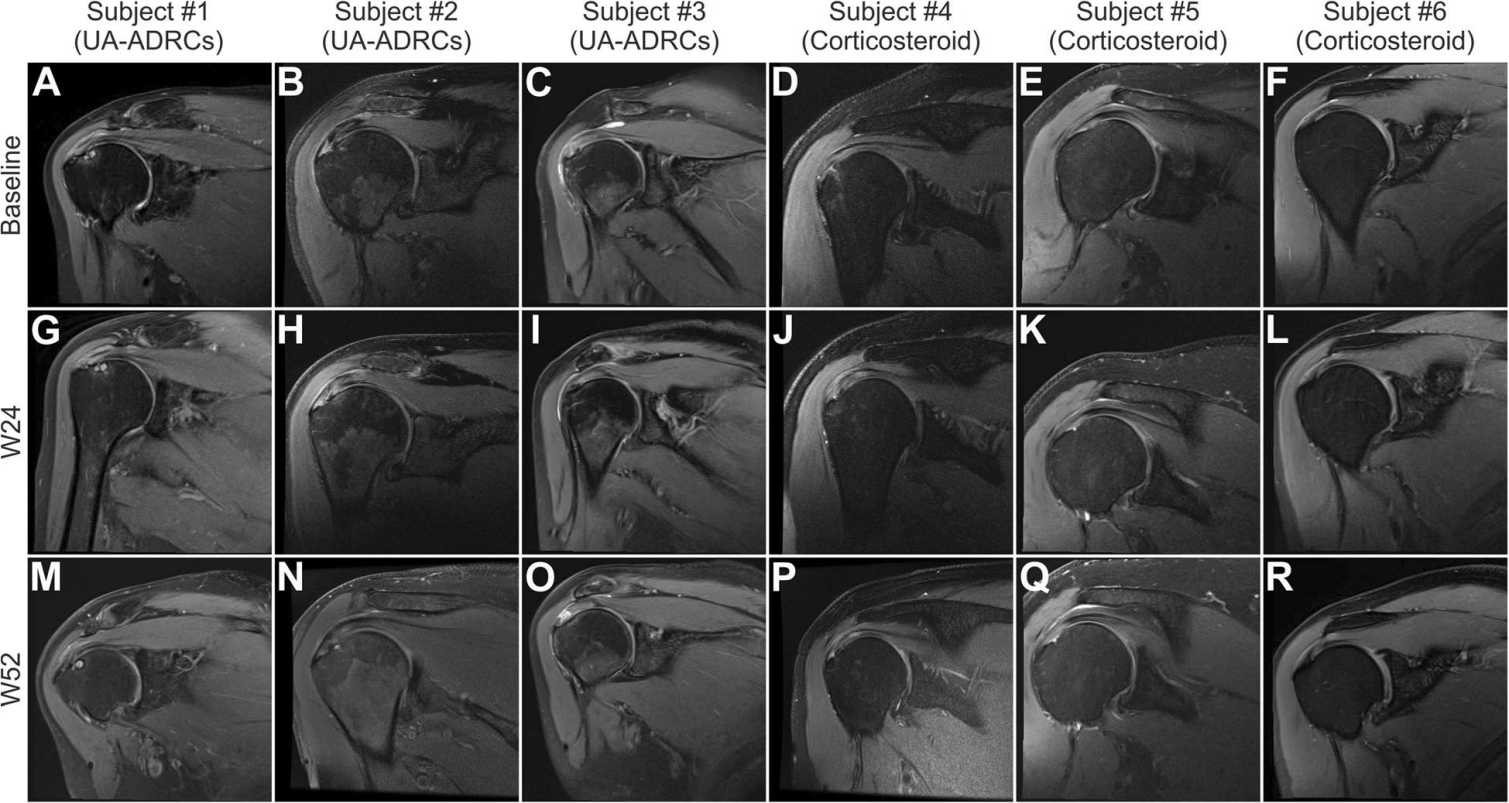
Coronal MRI scans (T2, proton density-weighted, fat-saturated (PD FS)) of the index shoulder of select subjects treated with respectively UA-ADRCs (a–c, g–i, m–o) or corticosteroid (d–f, j–l, p–r) in this pilot study. Repetition time (TR) was 2375 ms in n, 2950 ms in d, 3317 in j, 3500 in a, c, e, f, i, k, m, o, q, r, 3516 in p, 3660 in l, 3820 in g, and 3917 in b, h. Echo time (TE) was 34 ms in a, c, e, f, g, i, k–m, o, q, r, 37 in n, 45 in b, h, j, 46 in d, and 65 in p. NEX was 1 in a–m, o, q, r, 1.5 in p, and 2 in m
